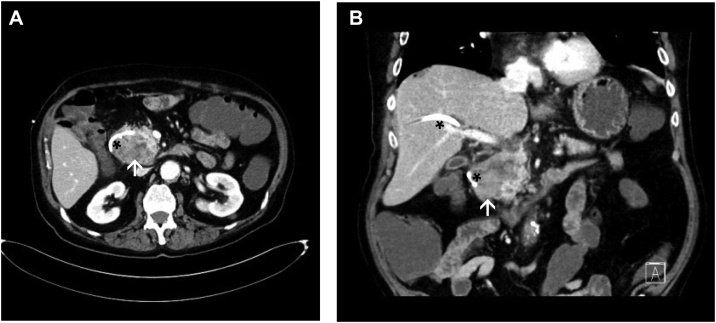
Computed tomography following PTCD. Control CT with adequate positioning of the PTCD (black asterisks) and relief of the bile duct system, and constant ill-defined tumor in the head of the pancreas (white arrows) (A transverse image, arterial phase; B coronal image, porto-venous phase).
